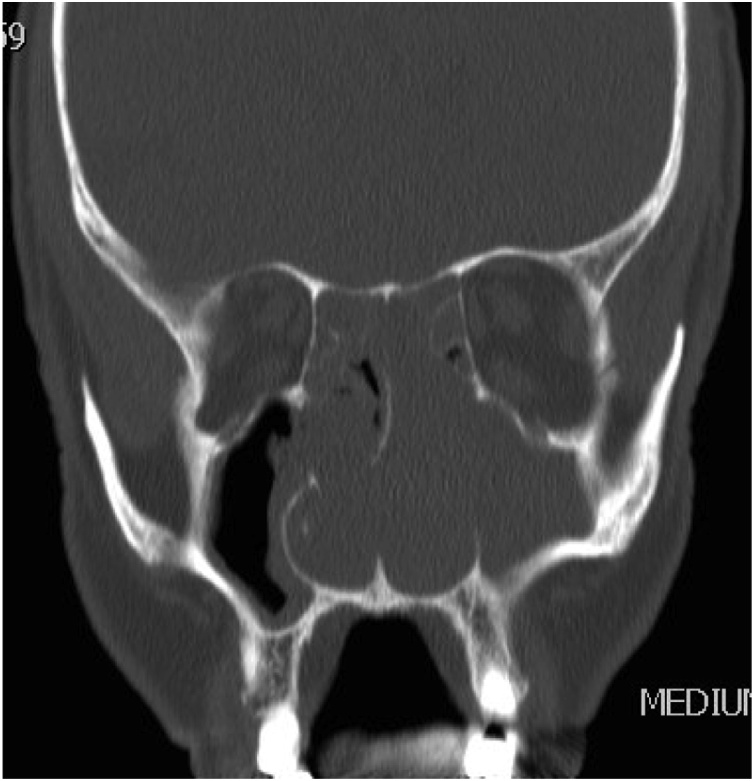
CT scan of the paranasal sinuses showed homogenous opacities filling both nasal cavities and the left maxillary, frontal and sphenoid sinuses.
